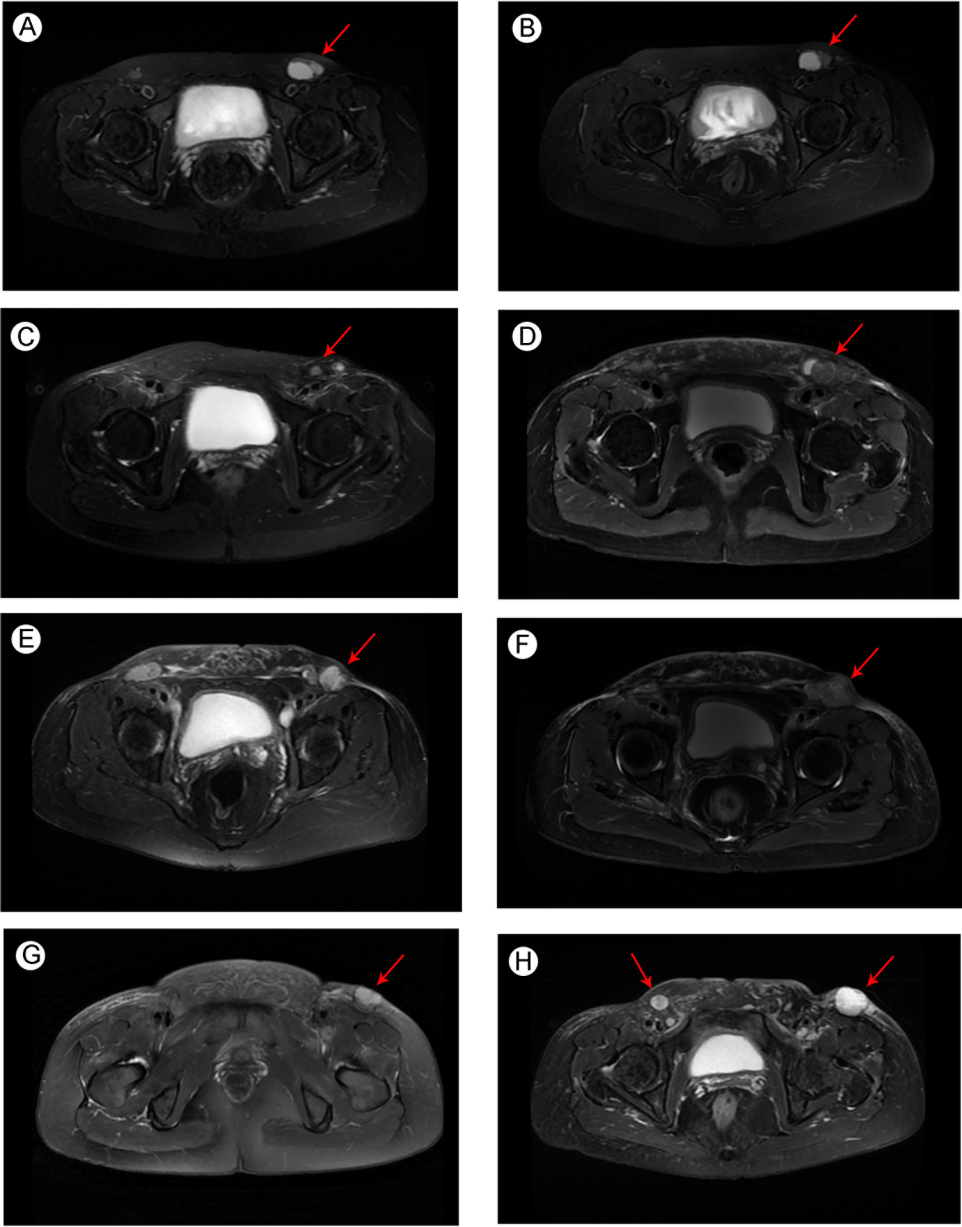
Pelvic MRI image of metastatic SGC (A) before docetaxel + nedaplatin treatment in October 2018; (B) after docetaxel + nedaplatin treatment in February 2019; (C) after the radiotherapy in September 2019; (D) before albumin-bound paclitaxel + camrelizumab treatment in December 2019; (E) after albumin-bound paclitaxel + camrelizumab treatment in September 2020; (F) before pemetrexed + nedaplatin + camrelizumab treatment in December 2020; and newly developed metastatic lymph nodes (G) before pemetrexed + nedaplatin + camrelizumab treatment in December 2020; (H) after pemetrexed + nedaplatin + camrelizumab treatment in March 2021. Arrows indicate the tumors in the groin region.
